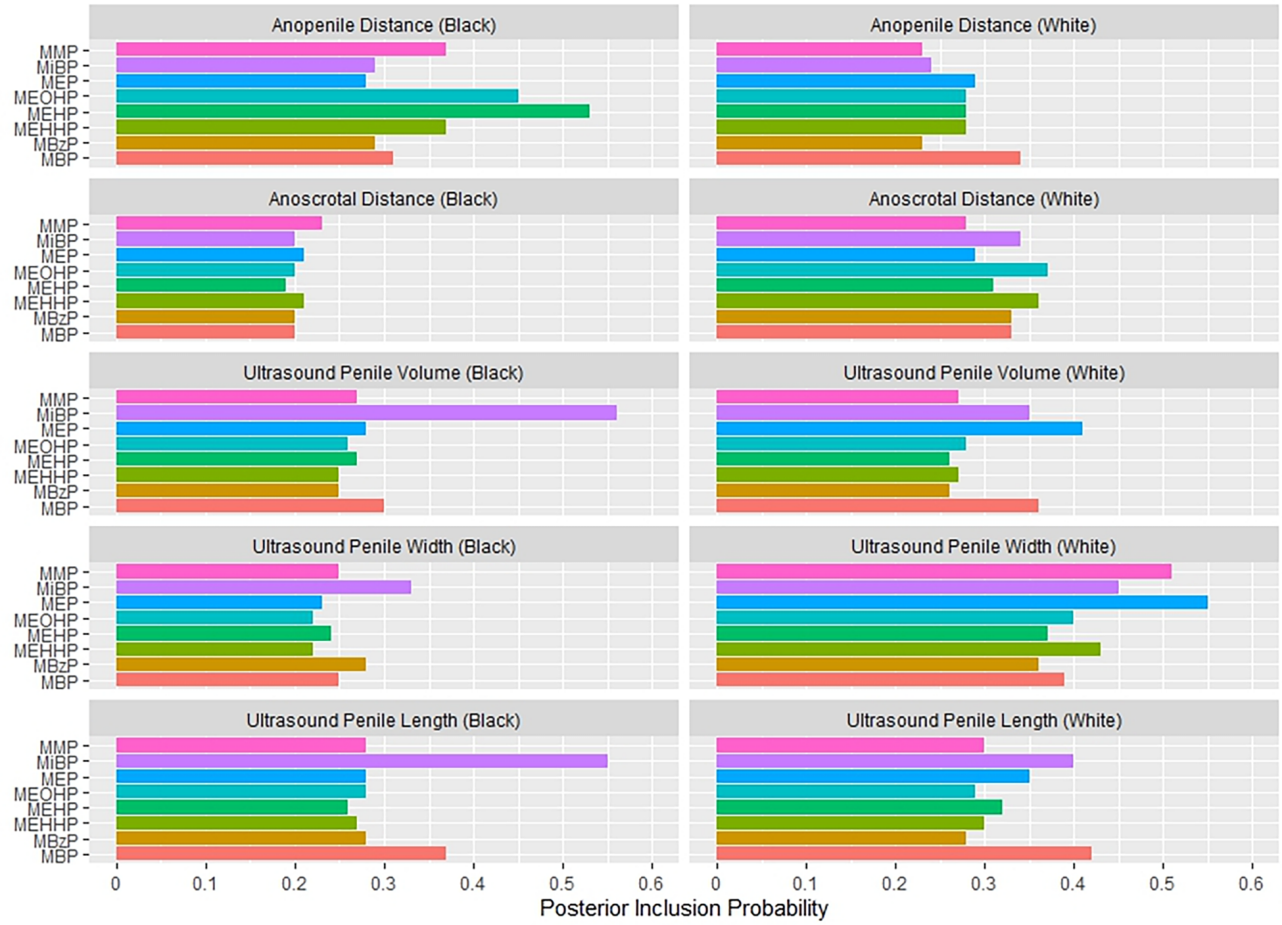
Posterior inclusion probabilities for urinary phthalate metabolite predictors of prenatal ultrasound penile dimensions and postnatal anogenital distance measures among male infants born to women in Charleston, South Carolina, by racial identity (*n* = 179).
